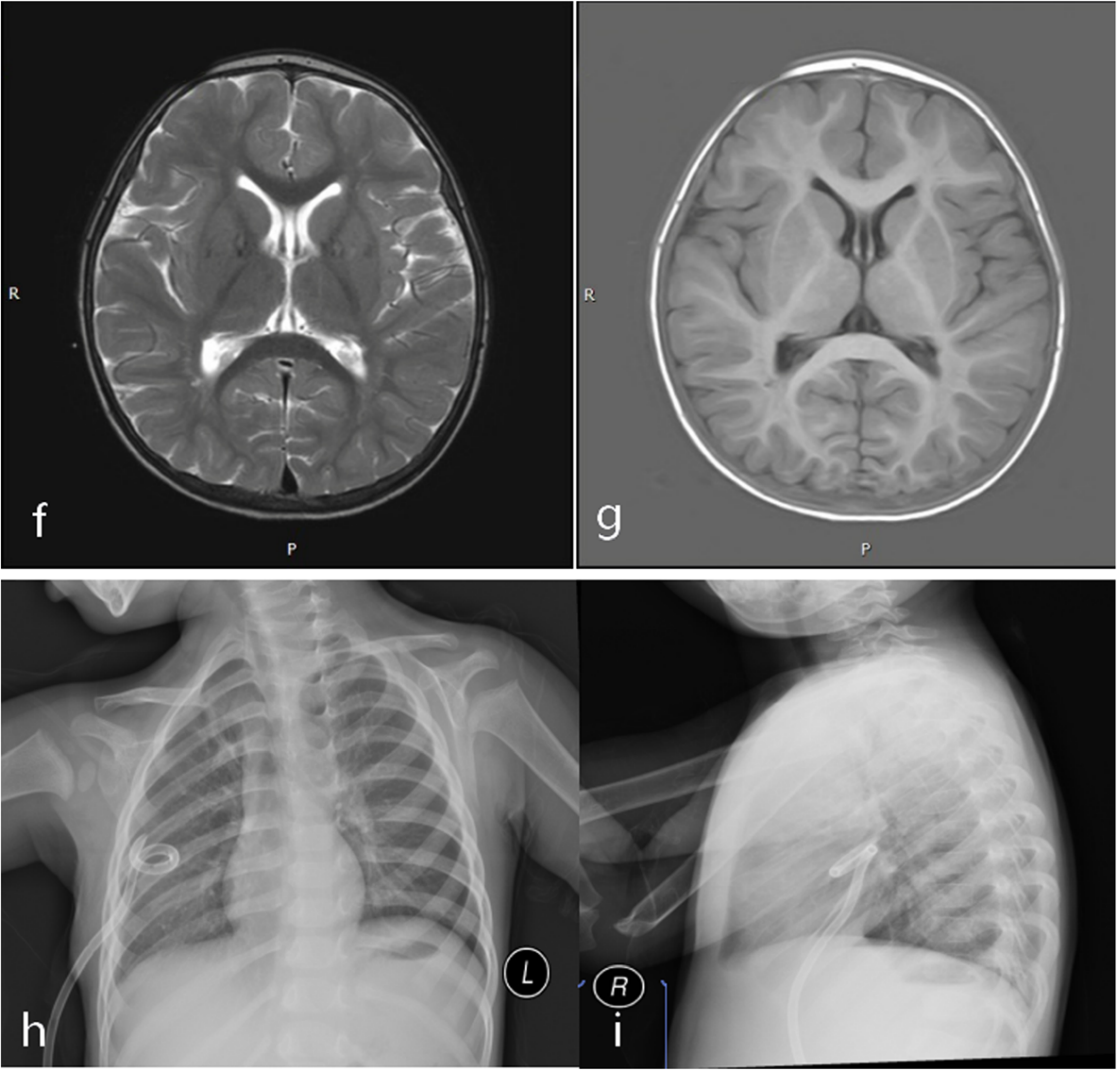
f–i Chest X-ray and brain MRI. f, g The MRIs show no ventriculomegaly or paraventricular edema after removal of cisternal drainage. h, i The chest X-ray show the no effusion in the thorax
